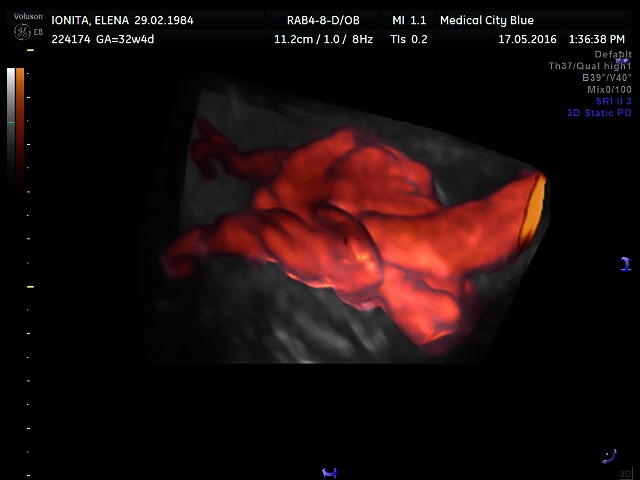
True umbilical cord knot on 3D power Doppler Glass Body
